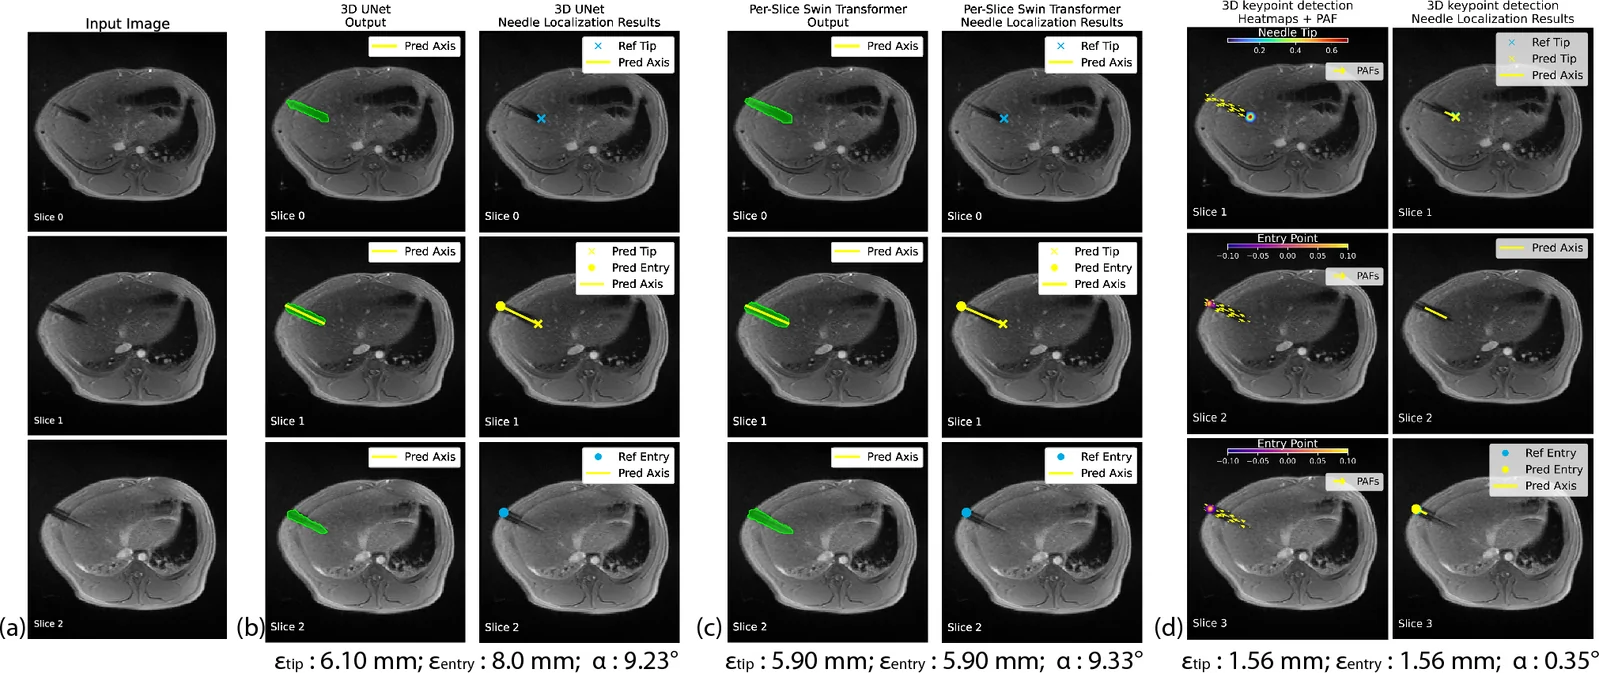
Example of needle localization on a multislice controlled-breathing (MS-CB) MR image set a using 3D UNet b, Per-slice Swin Transformer c, and the 3D keypoint detection network d. The needle axis was shown as a disconnected line if the needle axis crossed multiple slices
